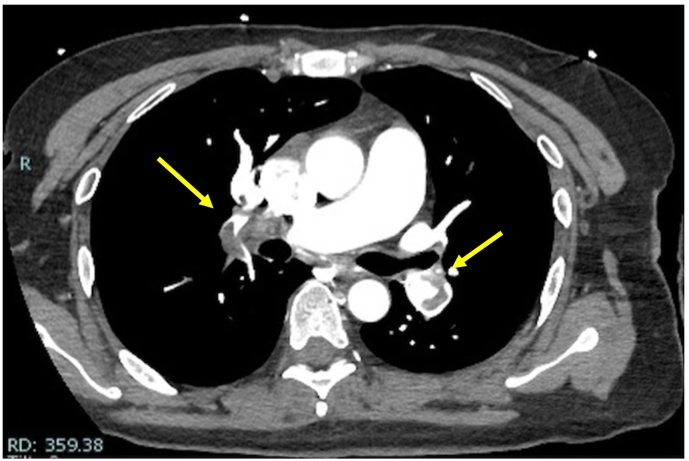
Computed tomography at admission. Yellow arrows indicate the thrombi.
